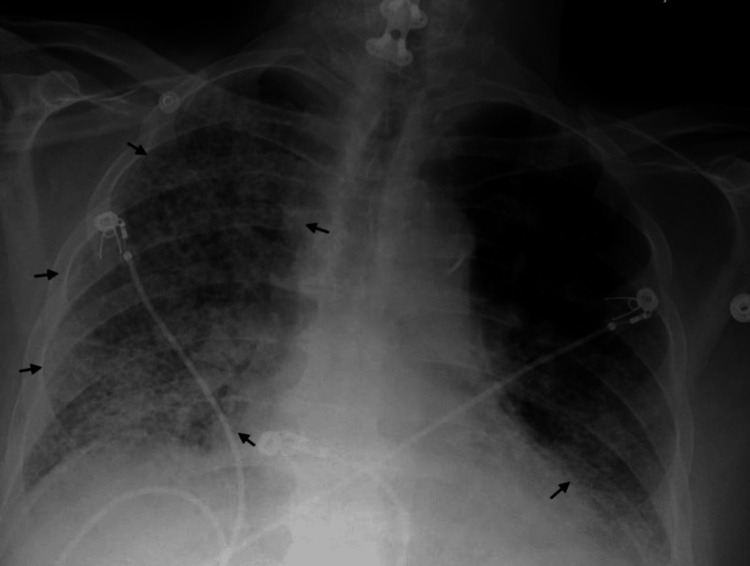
PA view CXR on admission CXR on admission demonstrating a mixed reticular and consolidative pattern throughout both lungs (black arrow), more pronounced in the right hemithorax. CXR, chest X-ray
